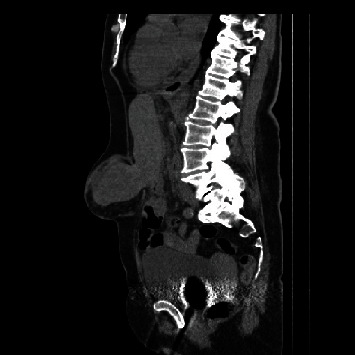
Sagittal section of noninjected CT abdominal scanner demonstrating herniation of liver segment III through the *linea alba*.
